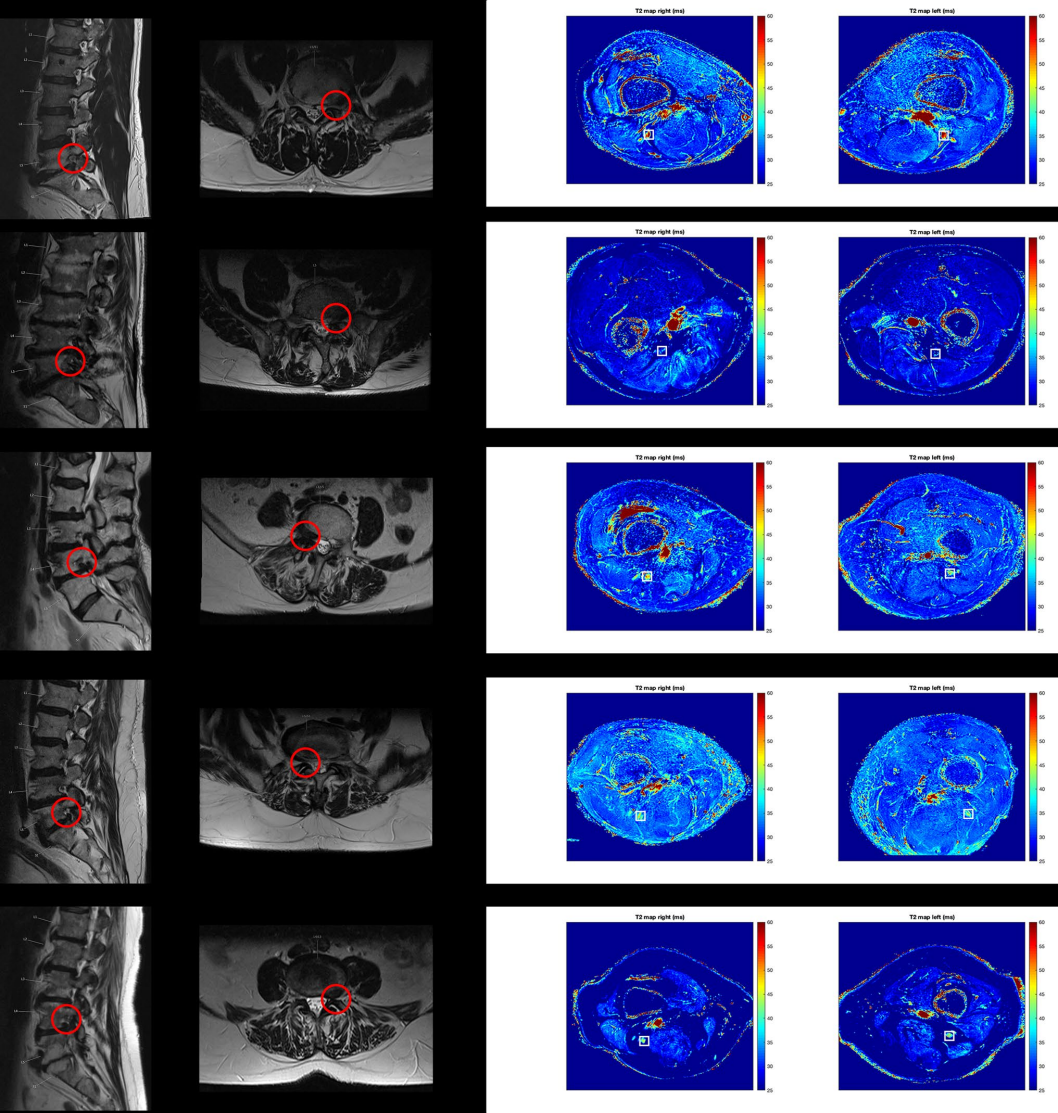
Case series of patients. Sagittal and axial slices of structural magnetic resonance imaging (MRI) from a case series of five patients with unilateral sciatica due to degenerative disc disease with disc herniation and unilateral nerve contact of degenerated disc material (red circles). Furthermore, representative axial T2 maps derived from the three-dimensional (3D) T2-prepared turbo spin echo (TSE) sequence are shown for the right and left lower thigh regions, depicting the distal sciatic nerve (white squares). Mean ± standard deviation of measured T2 values of the lower sciatic nerve was 61.5 ± 6.2 ms for the affected side and 43.3 ± 2.4 ms for the contralateral, unaffected side
